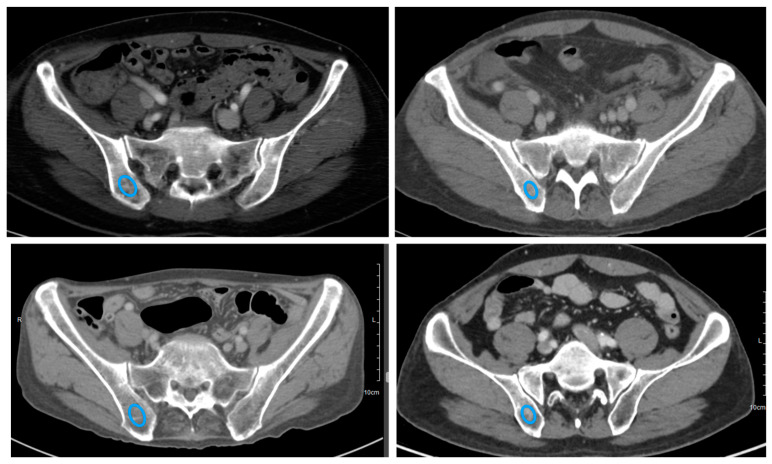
CT segmentation examples on contrast-enhanced axial scan for a patient with gastric cancer. Regions of interest were drawn in the right iliac bone on axial CT images (blue lines). Abbreviation: CT, computed tomography.
